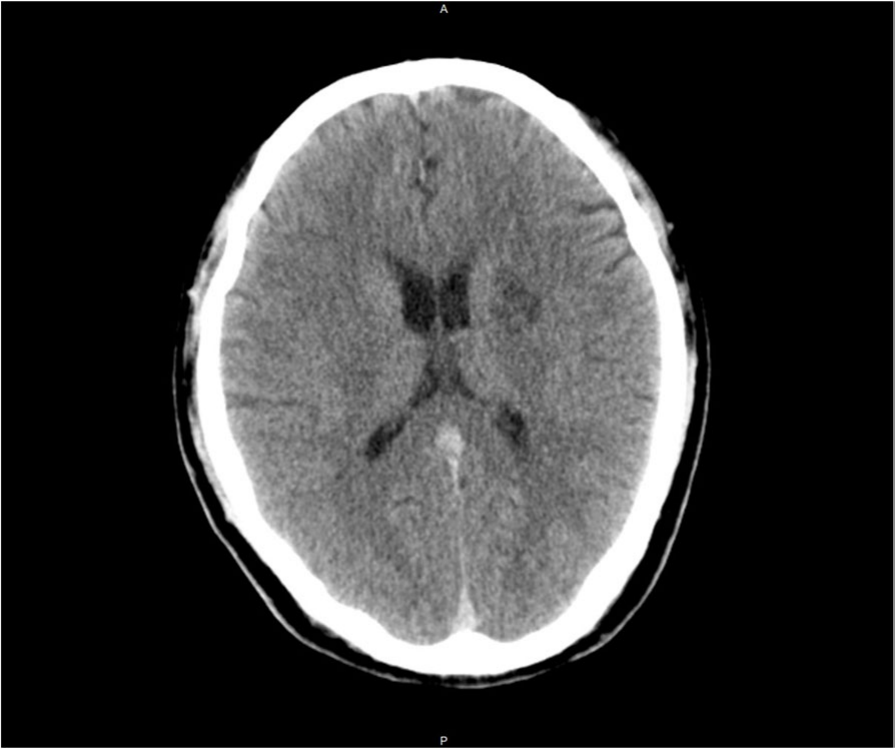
This is the patient’s CT imaging
